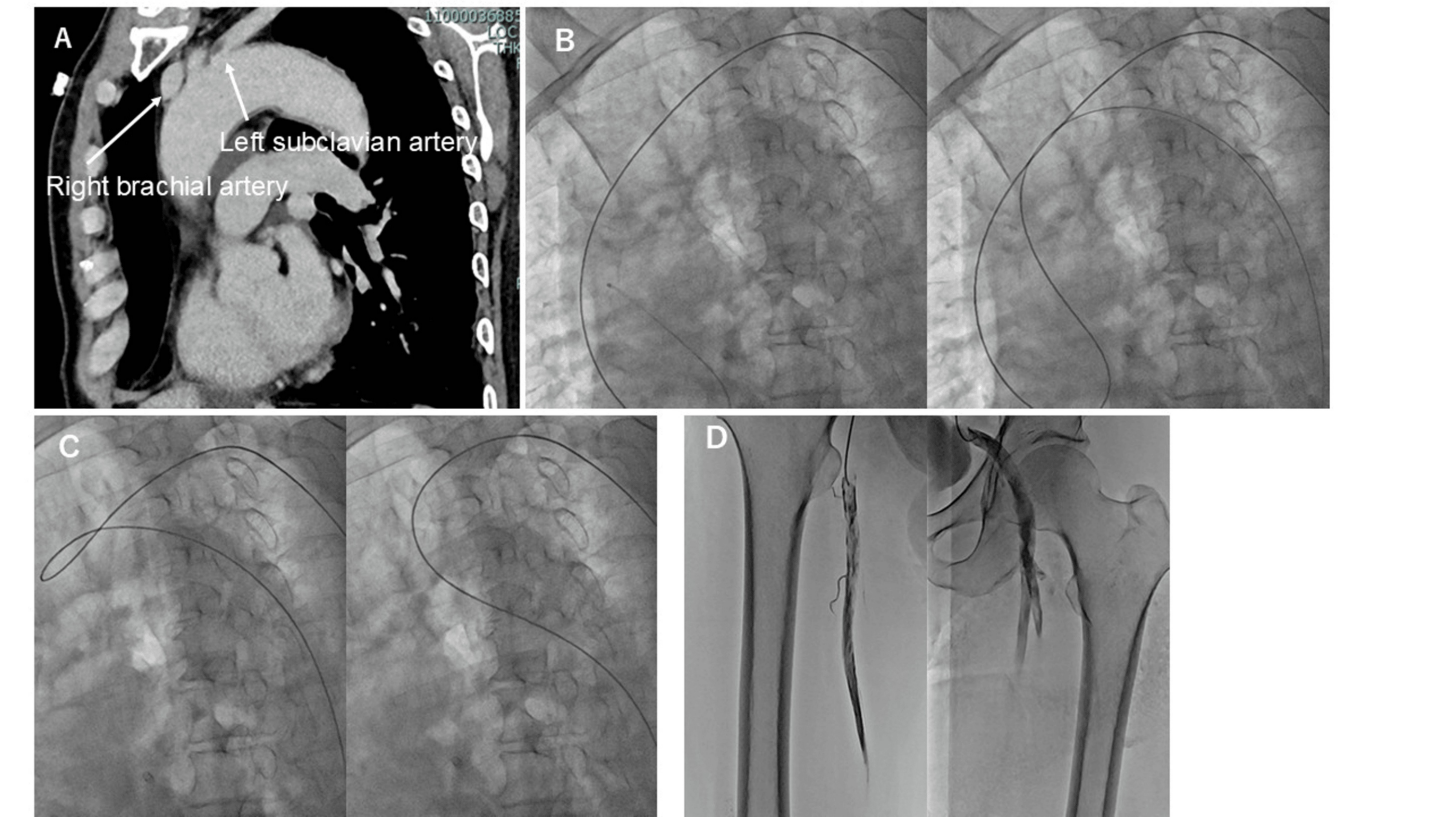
Lower limb angiography in Case 1. (A) The aortic arch is type III on contrast-enhanced computed tomography. (B) The 4-French Judkins right 4.0 catheter was manipulated (left figure) to advance a 0.035-inch guidewire into the descending aorta (right figure). (C) When the catheter was pulled up (left figure), the bend was removed, and the catheter was straightened (right figure). (D) The tip of the catheter was able to be advanced to the superficial femoral artery in the right lower limb (left figure) and to the external iliac artery in the left lower limb (right figure).
